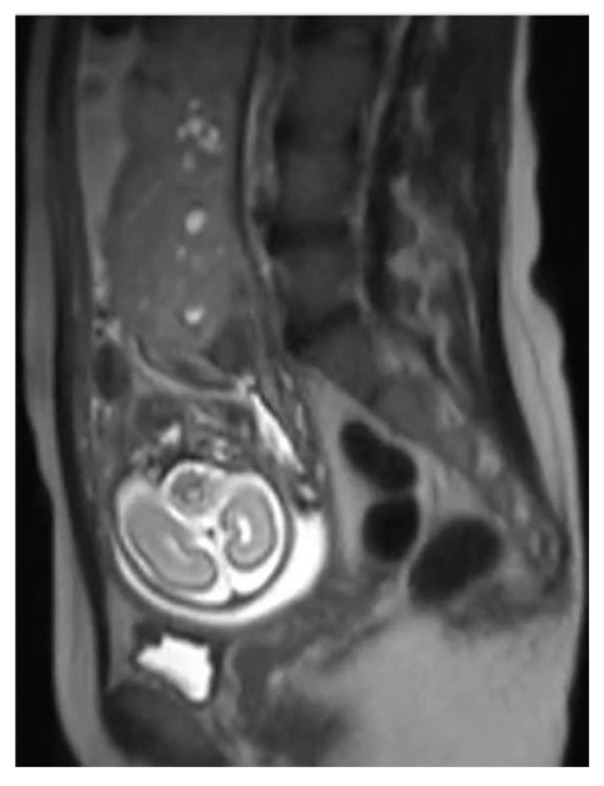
MRI scan showing placentomegaly, with no signs for abnormal adherence and the fetus head.
